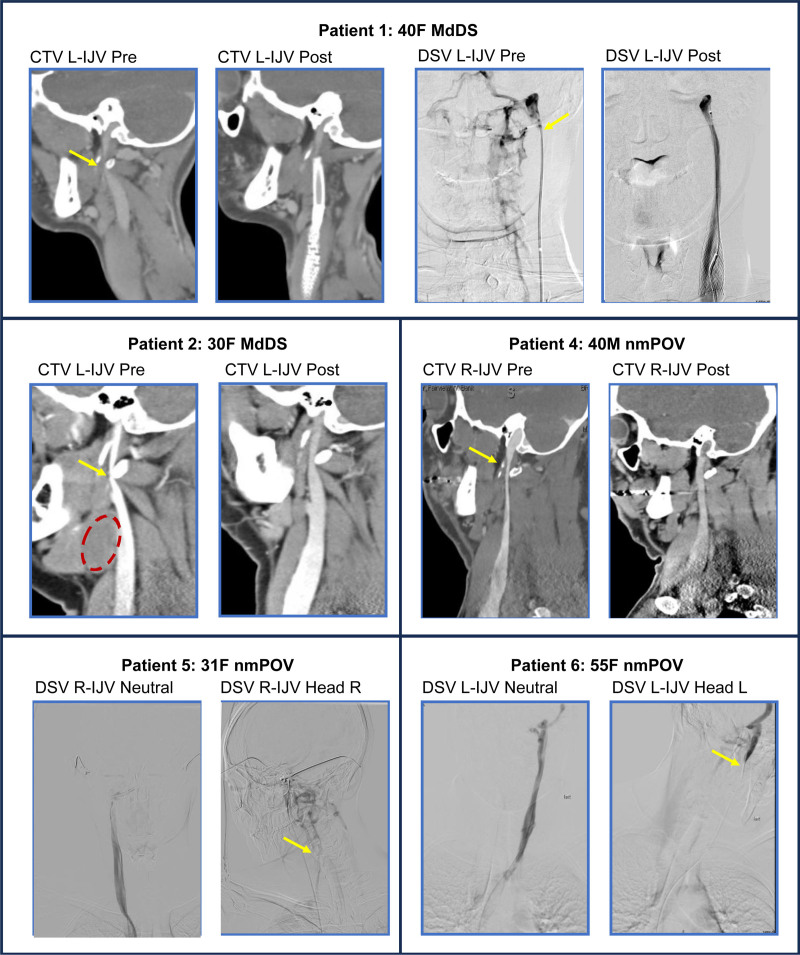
Imaging studies baseline and after treatment in select cases. Dotted oval in patient 2 encircles the branchial cyst, and yellow arrows indicate points of compression/obstruction. Patients 1, 2, and 4 had IJV compressions at C1. Patients 5 and 6 had muscular compressions of the IJV induced by head turning. All patients had concurrent TOS. CTV indicates computed tomography venogram; DSV, digital subtraction venogram; IJV, internal jugular vein; L, left; R, right; TOS, thoracic outlet syndrome.
